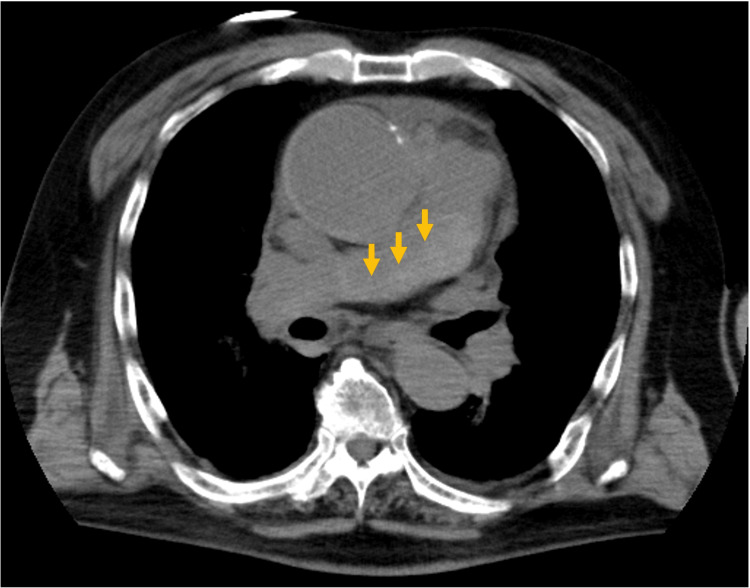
Non-contrast computed tomography of the right pulmonary artery main trunk On non-contrast computed tomography, a high-attenuation area is observed along the wall of the right pulmonary artery (yellow arrow).
